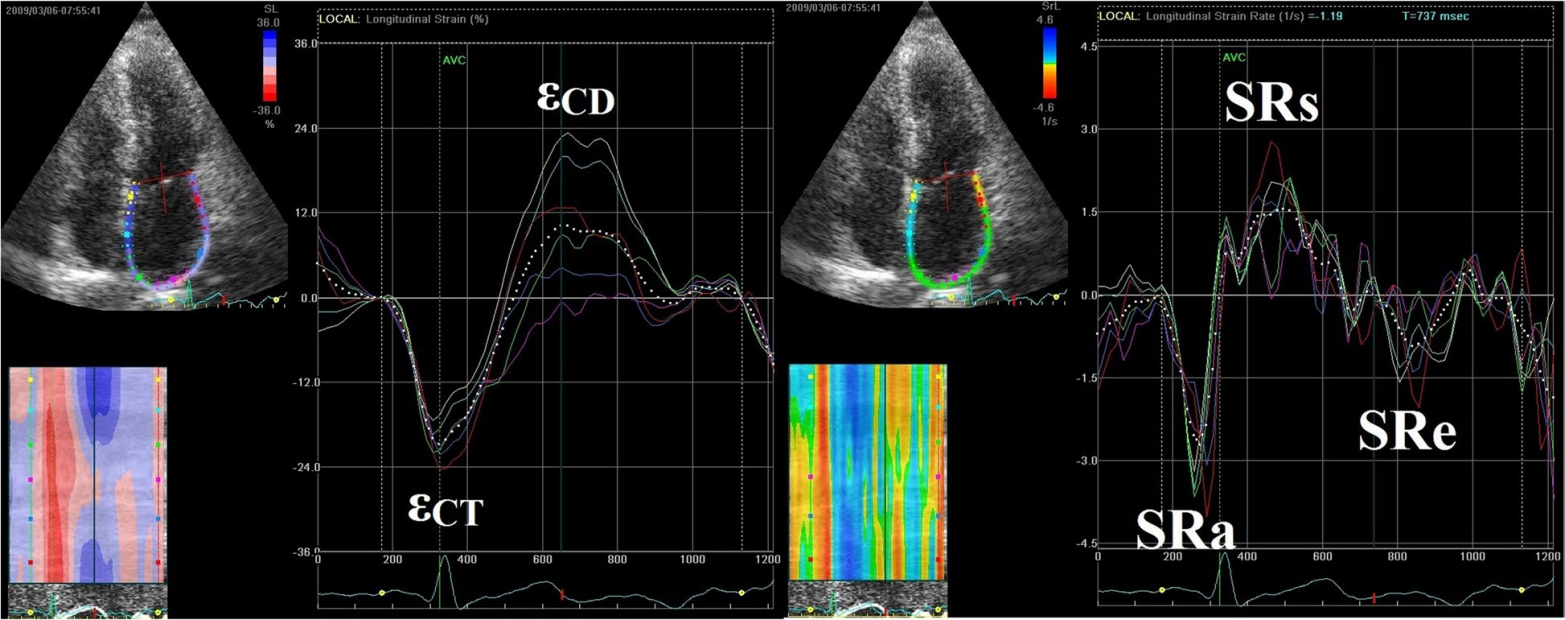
Left atrial strain and strain rate measured by speckle tracking imaging on apical four-chamber views
